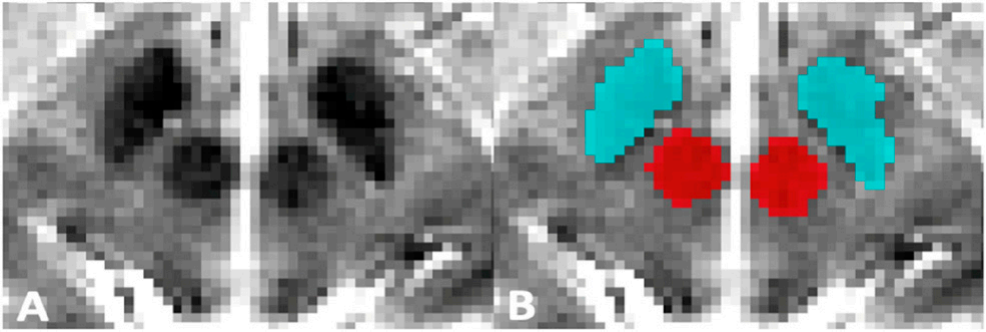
(A) Cropped midbrain region in an axial T2-weighted MRI, along with manual masks (B) for the SN-related T2 hypointensity (blue) and RN (red).
